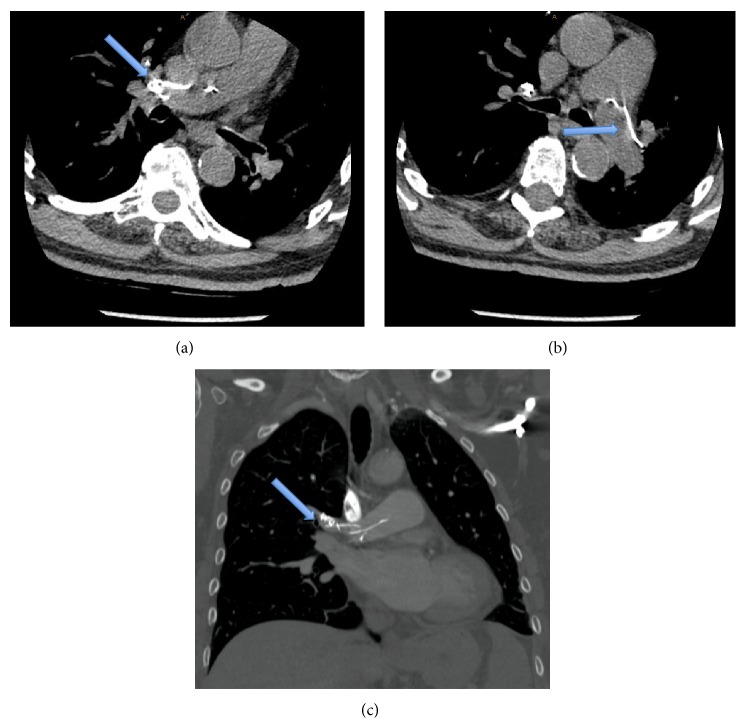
(a) An axial cut of the chest CT-scan of a nonenhanced CT chest showing multiple linear and extremely dense bone cement emboli involving pulmonary trunk and right pulmonary artery. (b) An axial cut of a nonenhanced CT chest showing a cement embolus involving the left main pulmonary artery. (c) A coronal cut of CT pulmonary angiogram also showing the cement emboli at the pulmonary trunk and right main pulmonary artery.
